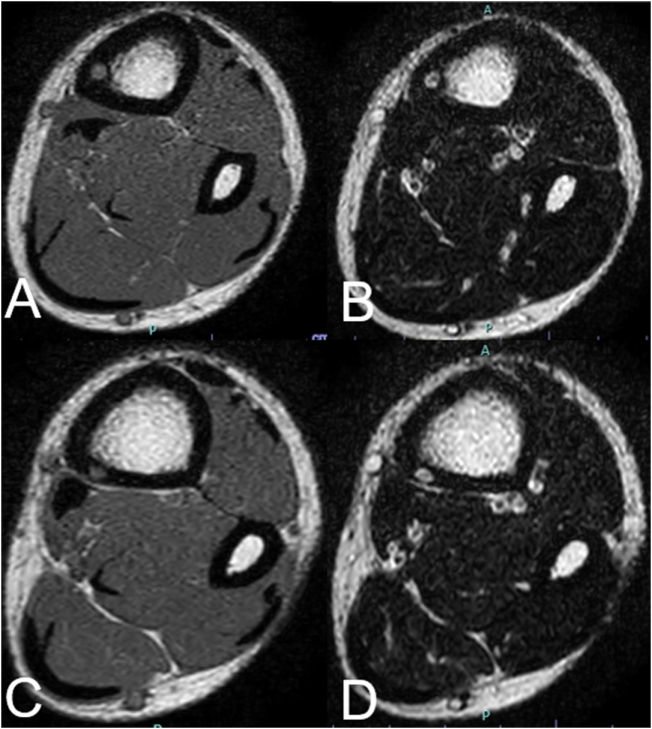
MRI showing isointense on T1-weighted images (A and C) and hyperintense on T2-weighted images (B and D).
